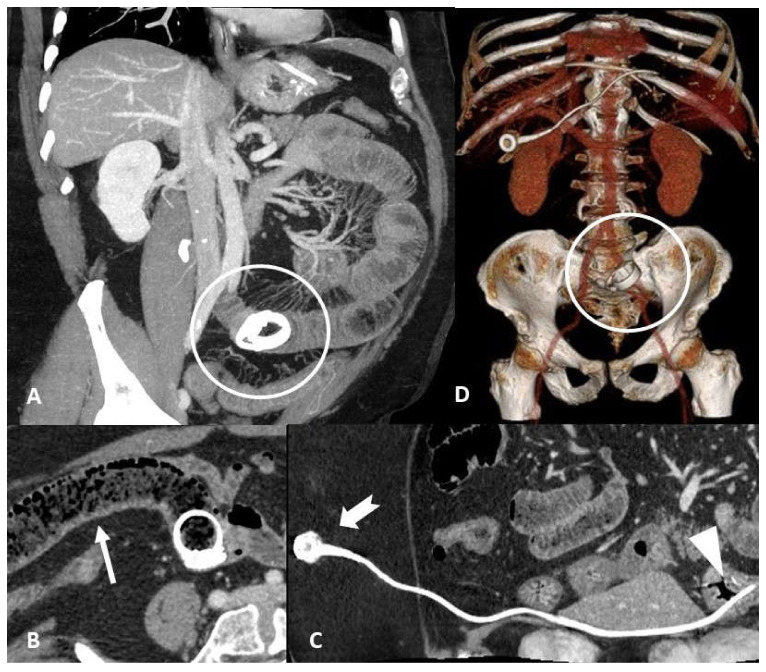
Obstruction. Sixty-four-year-old male with a 3-day history of abdominal pain, constipation, vomiting, and a history of surgery with sleeve gastrectomy and LAGB (laparoscopic adjustable gastric banding) insertion for morbid obesity. Contrast-enhanced CT maximum intensity projection images show (A) jejunum dilatation with transition point in mesogastrium caused by gastric band displacement; (B) marked dilation of the upstream loop by fecaloid material stasis (arrow) indicates mechanical obstruction. Contrast-enhanced CT multiplanar reconstruction scans (C) show port localization in the subcutaneous tissue of the anterior abdominal wall (notched arrow) and the tip of the connecting tube in the gastric cavity (arrowhead). CT 3D-reconstruction (D) better shows device disconnection, migration, and integrity of the two components.
